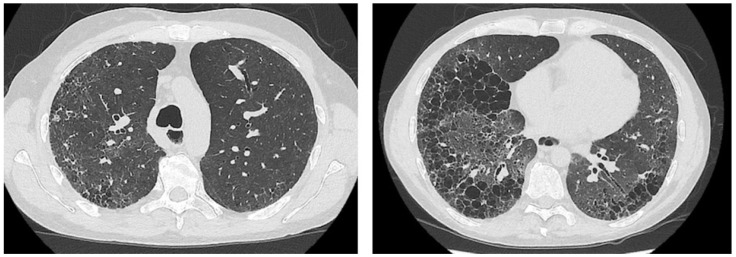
Axial High-Resolution Computed Tomography (HRCT) images showing a typical Usual Interstitial Pneumoniae (UIP) pattern that consists of predominantly basal and peripheral reticular opacities and honeycombing with traction bronchiectasis.
